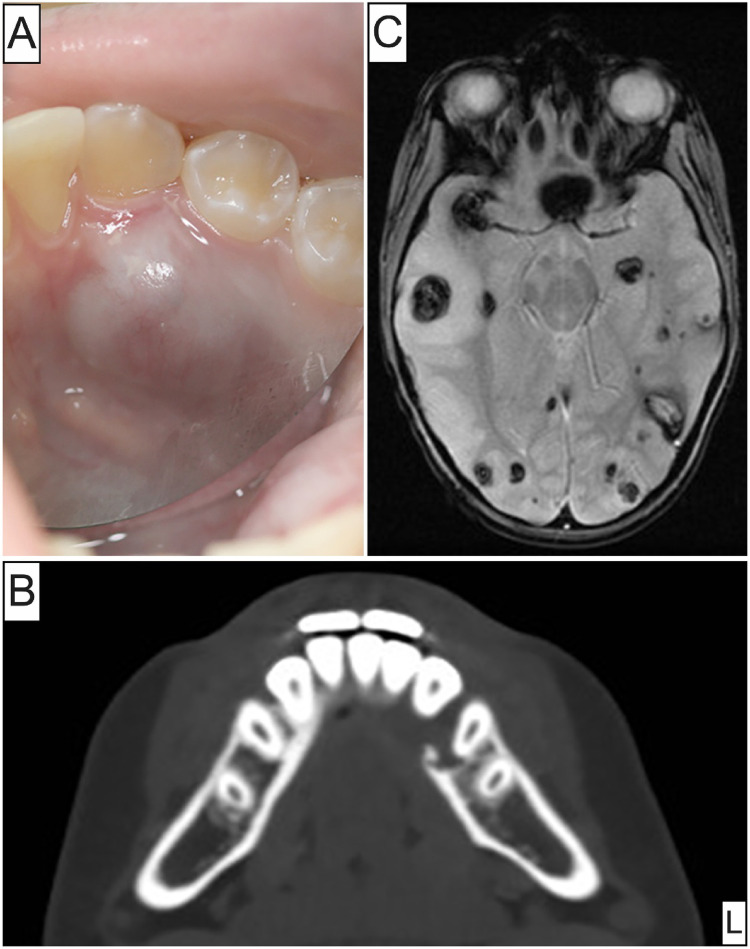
Clinico-radiologic presentation of Case 2. (A) Metastatic ES from retroperitoneal primary presenting as localized swelling of the lingual mandibular gingiva and alveolar bone; (B) Axial CT view revealing an expansile, destructive, osteolytic mass involving the left body of the mandible; (C) Axial MRI view of the brain confirming numerous ES metastases
